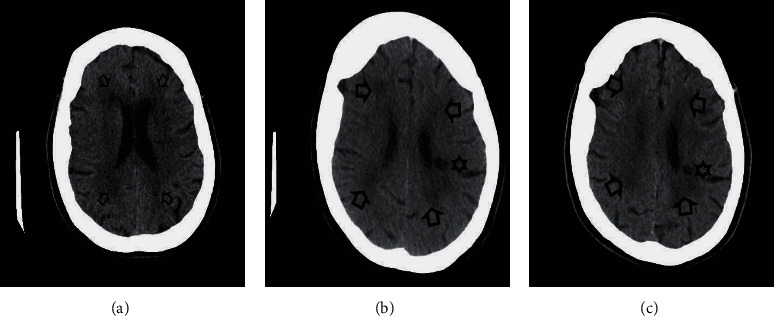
Axial noncontrast computed tomography of the head from 2017 to 2019 ((a) May 2017, (b) August 2018, and (c) October 2019). Key: arrow = hypoattenuation in the deep white matter is in keeping with moderate chronic small vessel ischaemic disease. Appearances are stable from 2017 to 2019. Star = chronic lacunar infarct in the left parietal lobe (first visualised in 2018).
